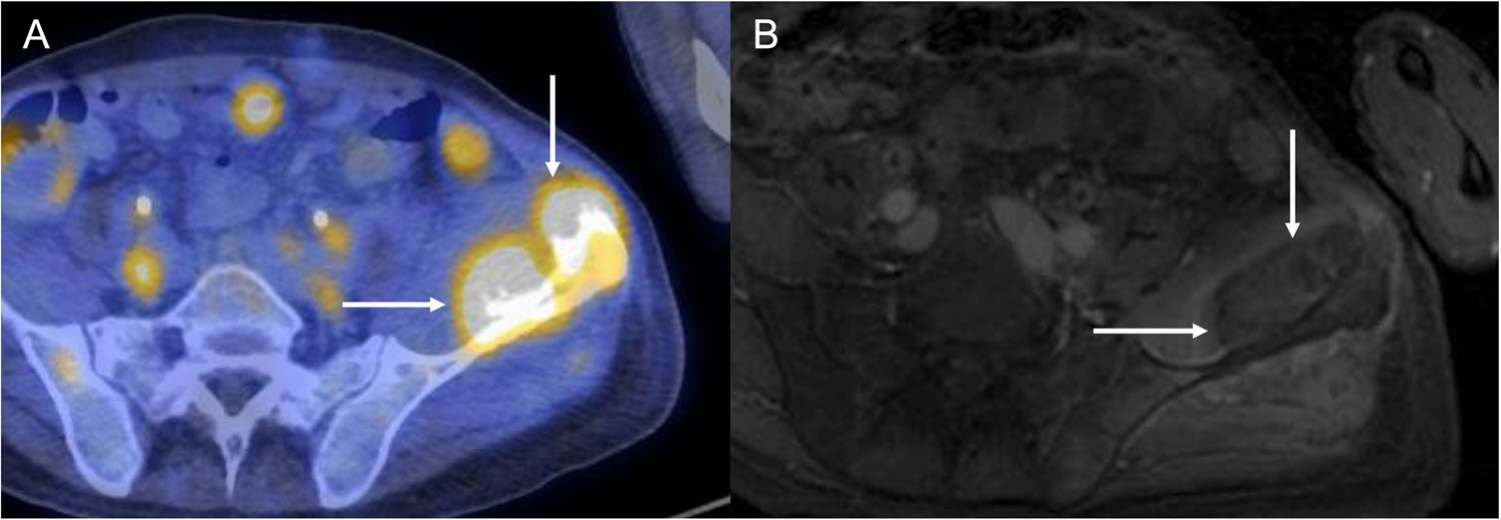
Osseous metastasis. A Axial fused pre-treatment FDG PET/CT of the left iliac bone in a patient with metastatic clear cell sarcoma demonstrating hypermetabolism (white arrows) within the bone and adjacent soft tissue. B Post-treatment axial T1 post-contrast images demonstrate relative hypoenhancement within the ablation area with minimal rim enhancement
